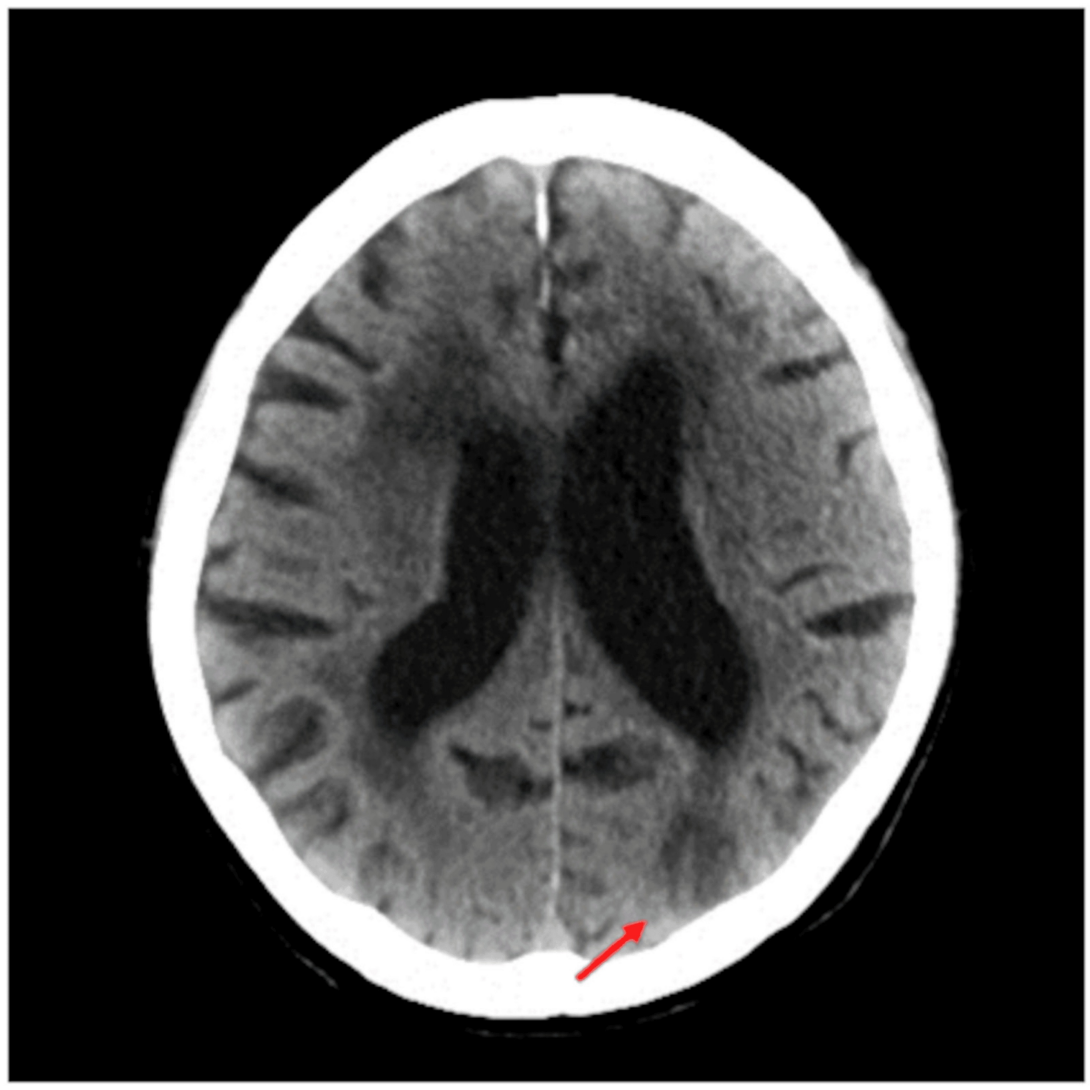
Computed tomography scan. A computed tomography scan revealed recurrent cerebral infarction with newly developed multiple low-density areas in the left parietal and occipital lobes (arrow).
